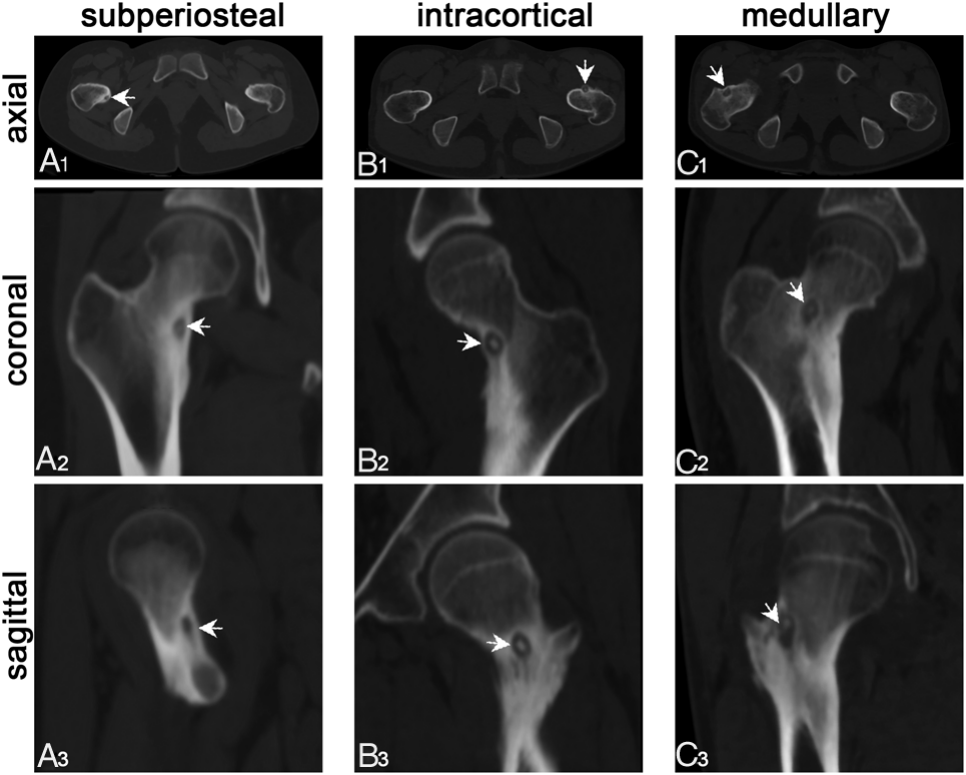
Oo was classified into sub-periosteal, intra-cortical, and medullary type based on the location of the nidus using thin-layer CT. (A1–A3) Sub-periosteal: the nidus is located under the periosteum and outside the cortex; (B1–B3) intra-cortical: the nidus is located inside the bone cortex and expands inwards and outwards; (C1–C3) medullary: the nidus is completely intramedullary. The white arrow shows the nidus, some of which have high-density calcification.
